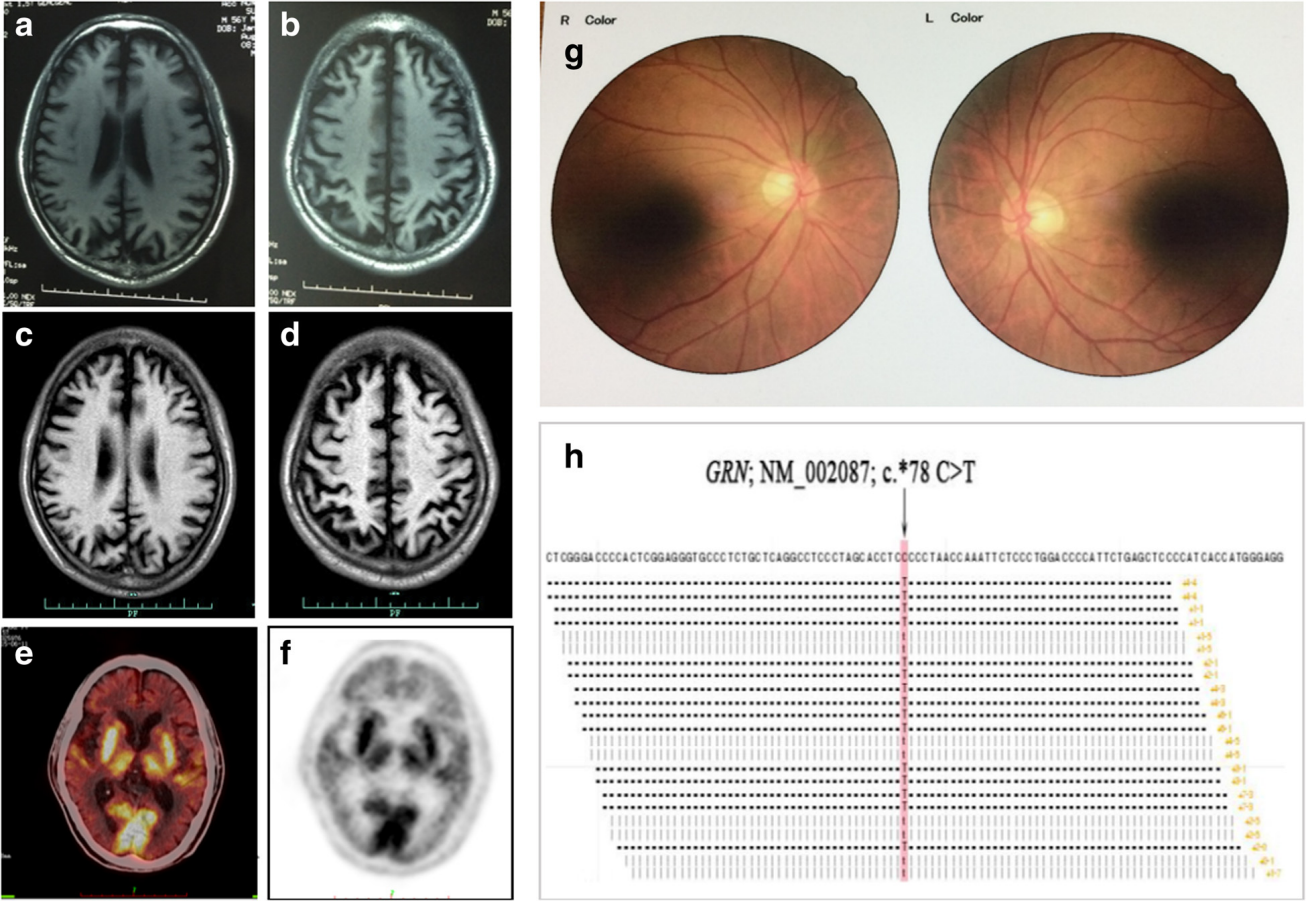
The clinical materials of the case. a-b Axial sections, T1-weighted sequences of MRI 4 years after disease onset (at 56 years of age) revealed cerebral atrophy, particularly in the posterior cortex. c-d One and a half years later, the secondary MRI revealed much more extensive atrophy within the frontal and occipital-parietal lobes, and bilateral partial parietal lobe. e-f 18F-FDG-PET showed diffuse bilateral hypometabolism in the frontal, parietal, temporal, and occipital lobes, particularly in the parieto-occipital regions. g Bilateral fundus examinations were normal. h Genetic testing showed a single nucleotide polymorphism rs5848 (c.*78C > T), located in the 3′-UTR of *GRN*
